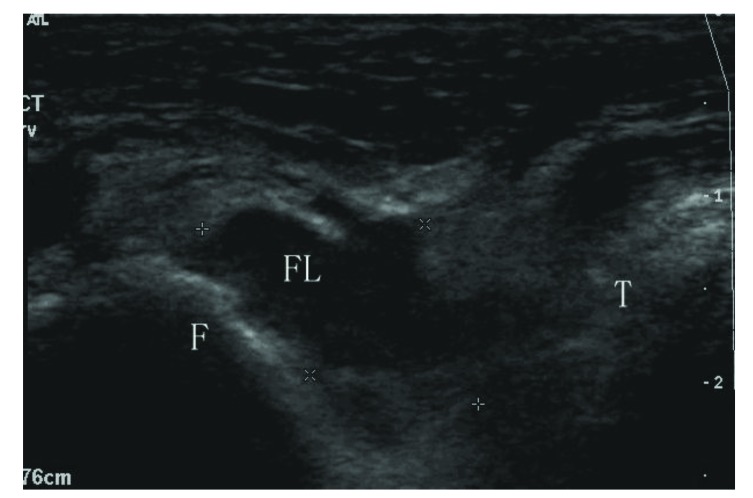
Sonogram shows the ligament fiber has been absorbed and could not be seen. (type V). F: fibula; FL: fluid; T: talus.
